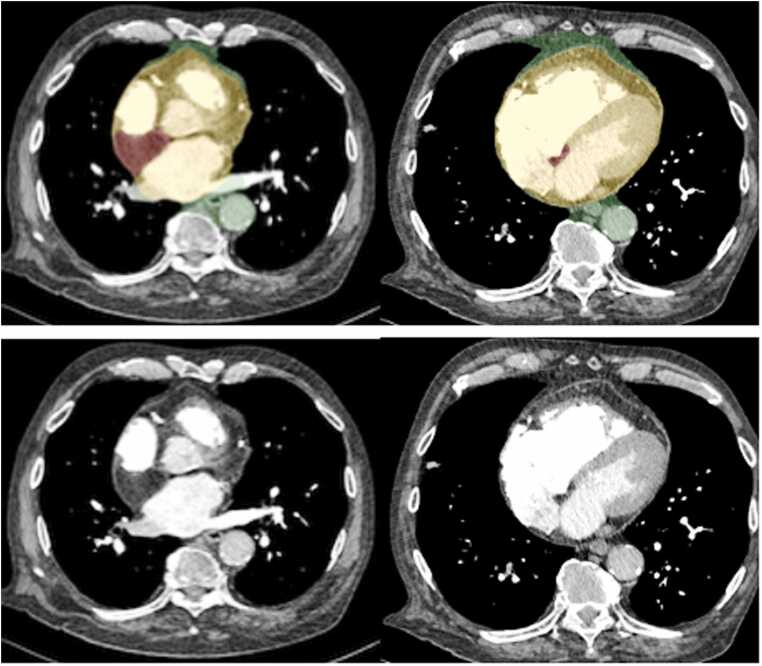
Exemplary case of segmentation in a 74‑year‑old man. Axial CT images at two different levels are shown. The lipomatous hypertrophy of the interatrial septum is highlighted in red, the cardiac structures (including the calculated epicardial fat tissue, EFT) are highlighted in yellow, and the mediastinal fat tissue (MFT) is highlighted in green. Note that a Hounsfield‑unit–based threshold from −190 to −30 HU was applied for fat volume quantification.
